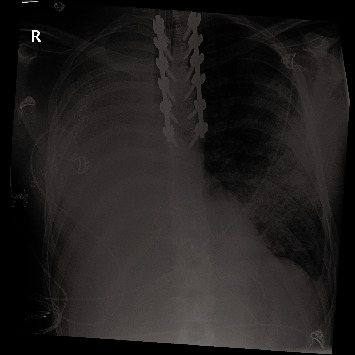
Chest radiograph on post-operative day #11.
